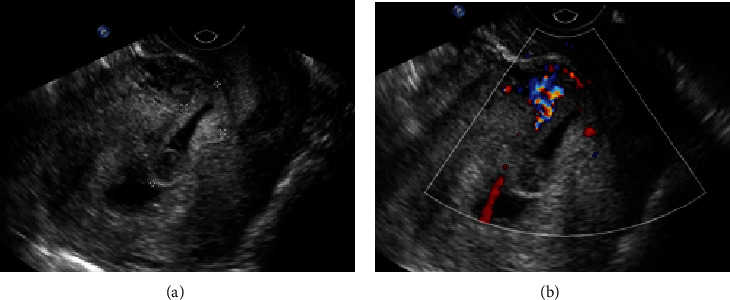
A 28-year-old female (gravida 4, para 2) presented with amenorrhea for 42 days. (a) The sagittal grayscale image showed that the sac was implanted in the lower endometrial cavity, protruding into the scar. There was a small amount of fluid in the uterine fundus. (b) The color Doppler image showed the trophoblastic blood flow from the lower anterior uterus. Chorionic villi were observed within the scar with a laparoscopy combined with uterine artery embolization.
